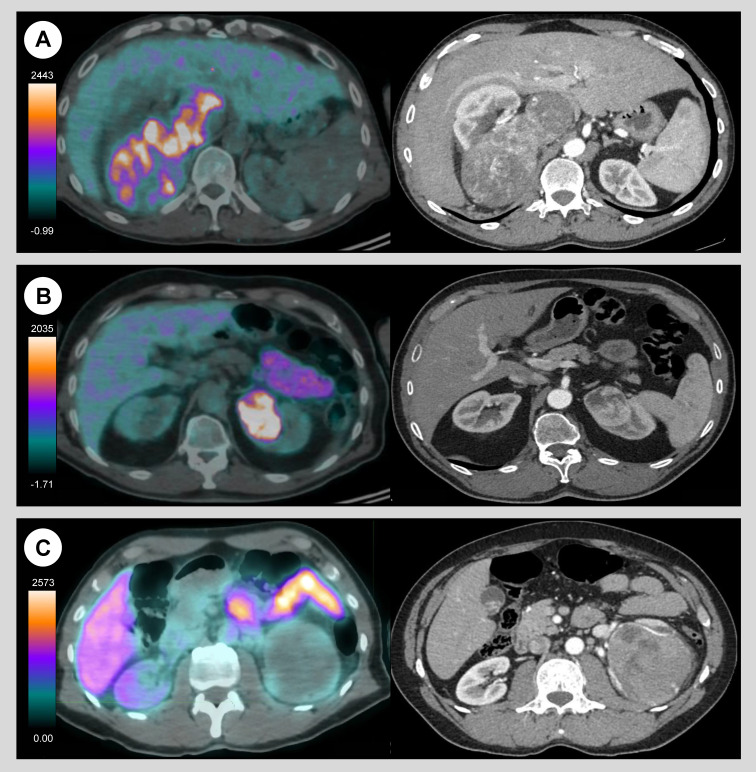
Axial *in-vivo* CAIX-PET/CT (left) and standard contrast-enhanced CT (right) images in three patients with high-risk renal cancer. CAIX-PET demonstrated significant albeit spatially heterogeneous uptake in the primary renal tumor in two cases -right kidney (A) and left kidney (B)- later confirmed as pT3a renal cell carcinoma at final pathology. In one case (C), CAIX-PET was negative, with no significantly detectable tumor uptake. This finding was confirmed at final pathology as a pT3a chromophobe tumor.
